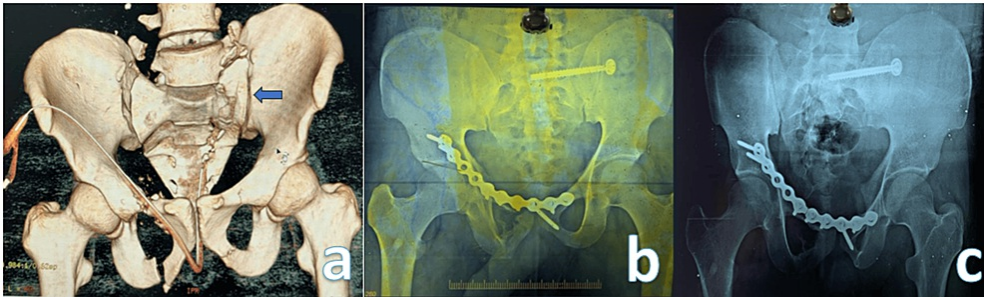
(A, B) Sacrum fracture fixation using a sacroiliac screw. (C) One-year follow-up X-ray.
